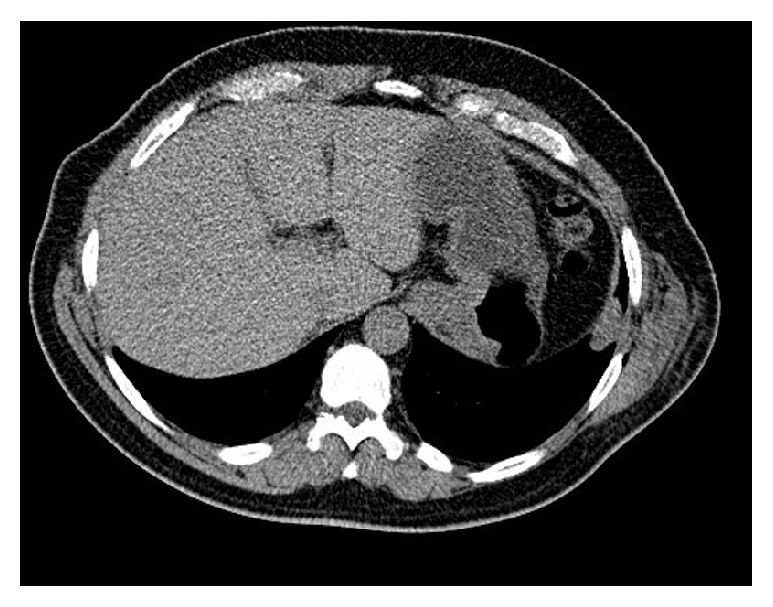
Chest CT-scan shows a 1.8 cm left lower lobe nodule.
